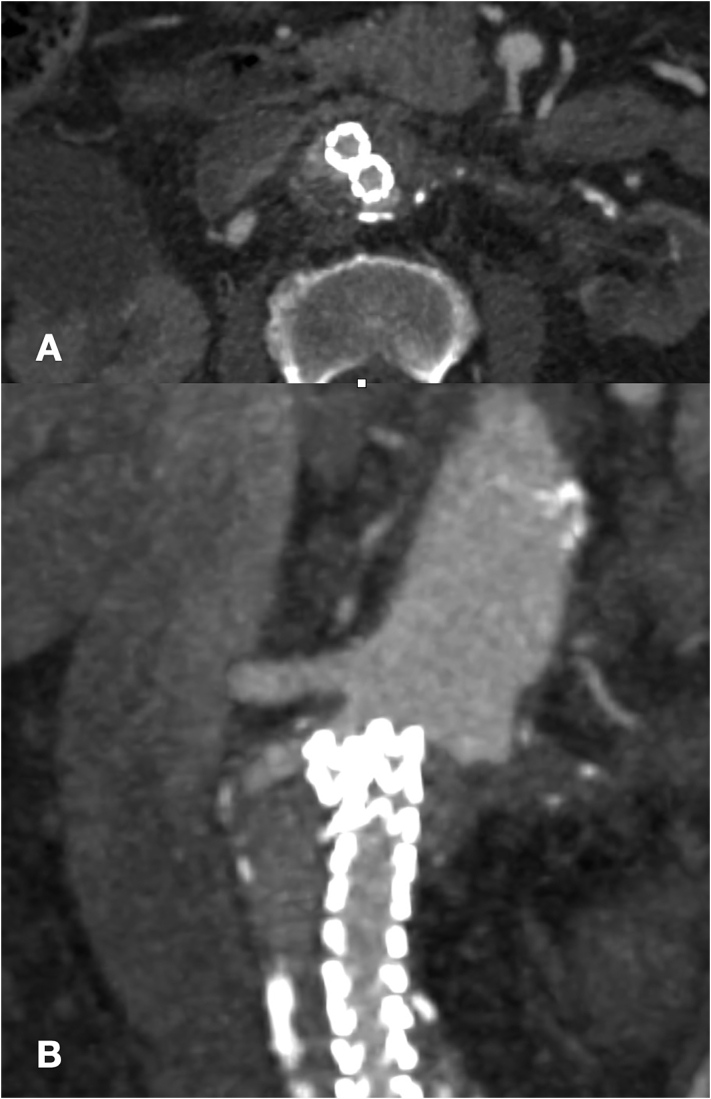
Preoperative CT Angiography showing endoleak in traversal plane (A) and coronal plane (B).
